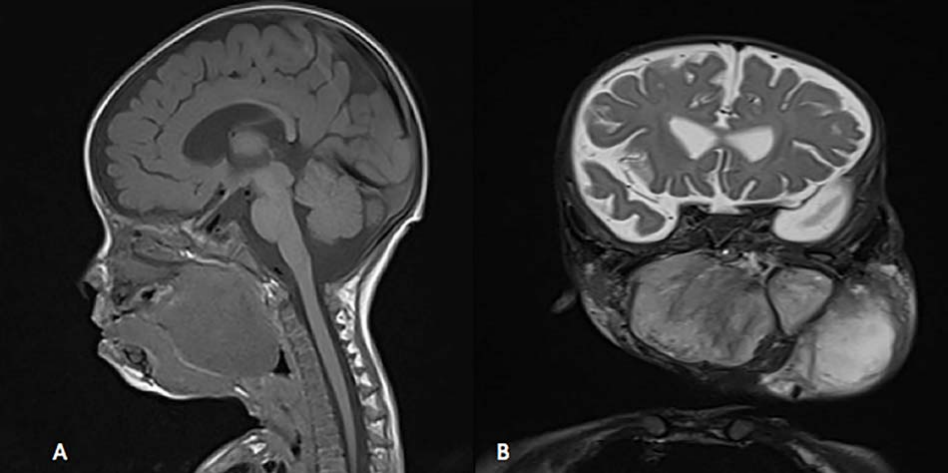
MRI, (A) sagittal view, showing the mass with extension into pharynx and oral cavity pushing the tongue base, (B) coronal view, the mass involving the neck, parapharyngeal space and pharynx up to base of skull.
